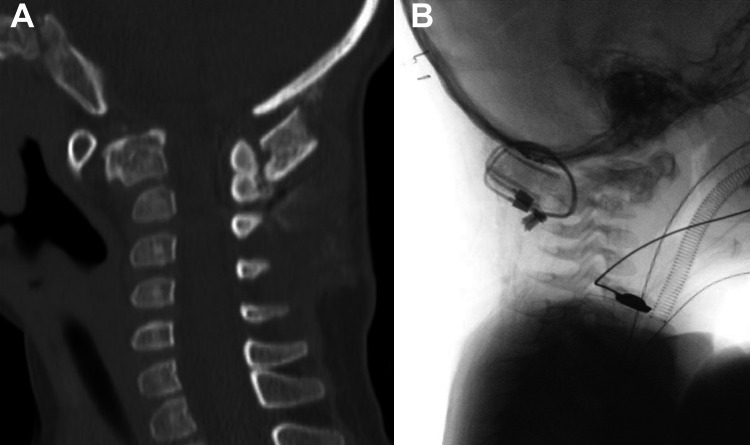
Postoperative. (A) Neck CT showing interval wire fusion of the occiput and upper cervical spine with the significantly improved caliber of the spinal canal and reduced spinal cord compression. (B) Craniocervical junction fluoroscopy demonstrating interval posterior stabilization of the skull base and C1-C2 posterior elements by cerclage wires to correct for the atlantoaxial instability.
